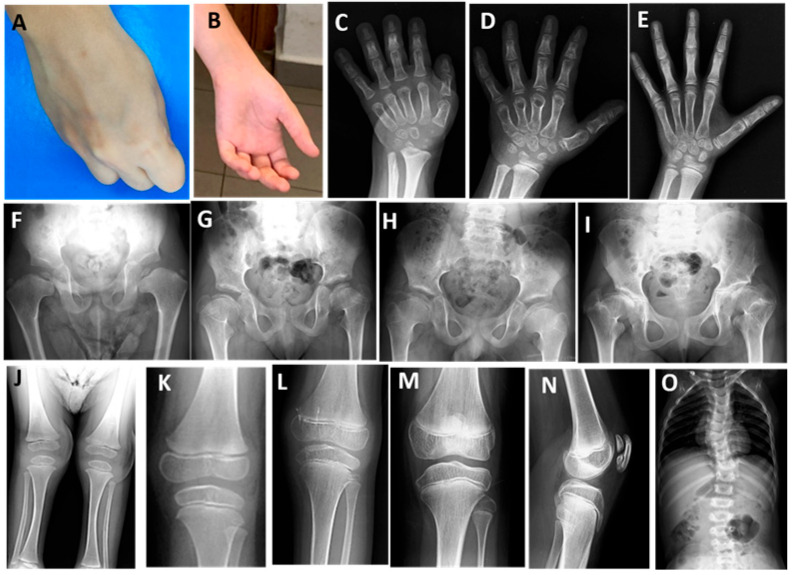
Patients with MED4. P15 at 11 years (A,I,M,N) and 5 years (E), P16 at 7 years (B,H,L), P17 at 3 years (D) and 5 years (G,K), and P18 at 2.5 years (C,F,J,O). Note the flexion contracture of the fingers (A,B), advanced bone age (C–E), metaphyseal irregularity (C), and shortening of the metacarpal bones (D). Coxa vara with broad, short femoral necks and small femoral epiphyses (F), flat and crescent-shaped femoral epiphyses with broad and short femoral necks (G,H,I) and protruding trochanter were revealed on pelvic radiographs (I). Note the short lower limb (J), broad metaphyses and flattened knee epiphyses (K–M), the classic appearance of a ‘double-layered patella’ on the lateral radiograph of the knee (N), and scoliosis (O).
